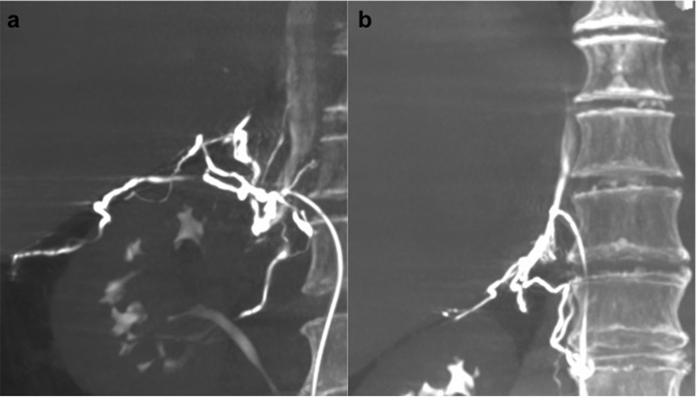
A 57-year-old man with primary aldosteronism and bilateral adrenal nodules. (a) On Dyna computed tomography maximum intensity projection imaging in the coronal plane, the renal capsular vein was opacified with the venous orifice at the T11/12 intervertebral level. (b) After adjustment of the catheter position, the right adrenal vein was opacified with the venous orifice at the mid T11 vertebral level.
